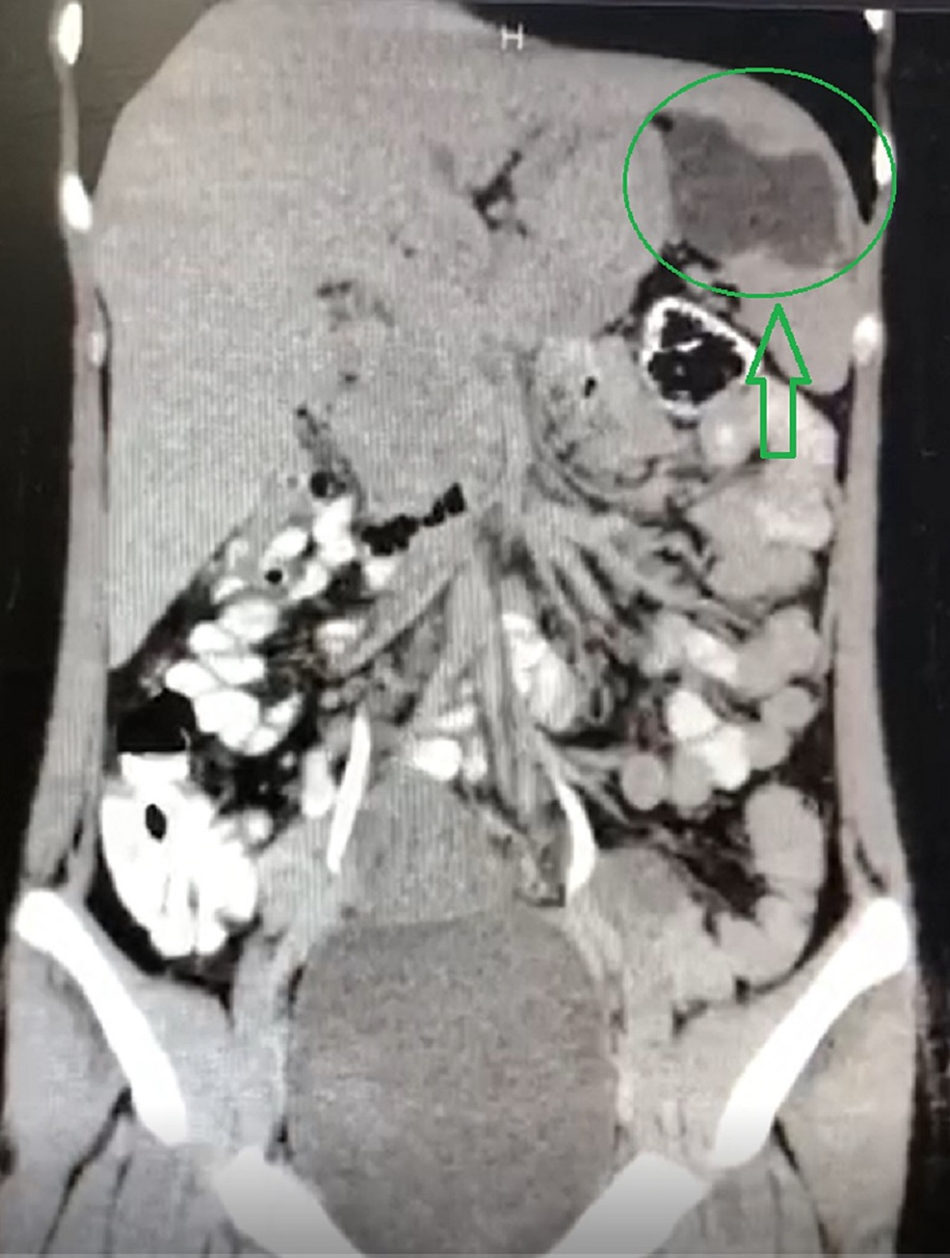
Coronal view of splenic hydatid cyst on abdominal CT scan The green arrow shows the location of the splenic hydatid cyst
